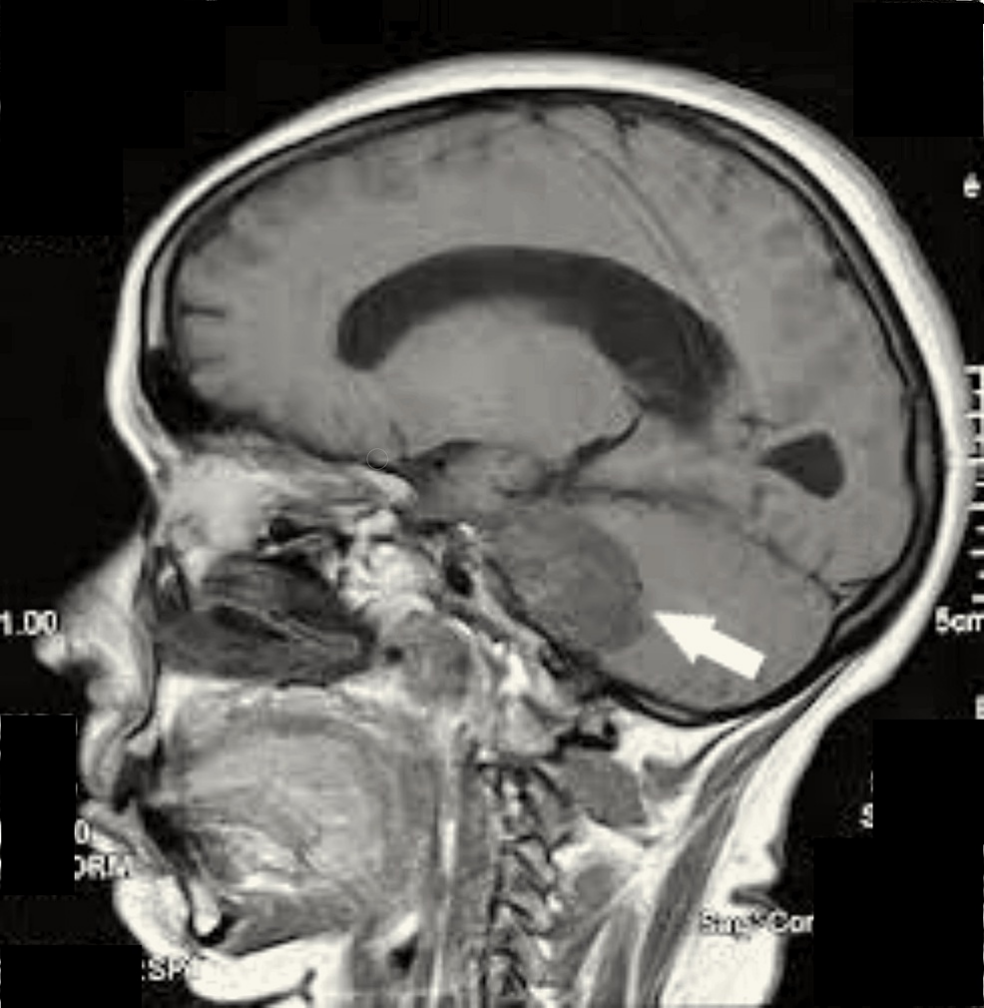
MRI Brain (sagittal section) showing the hypointense lesion compressing the cerebellum
